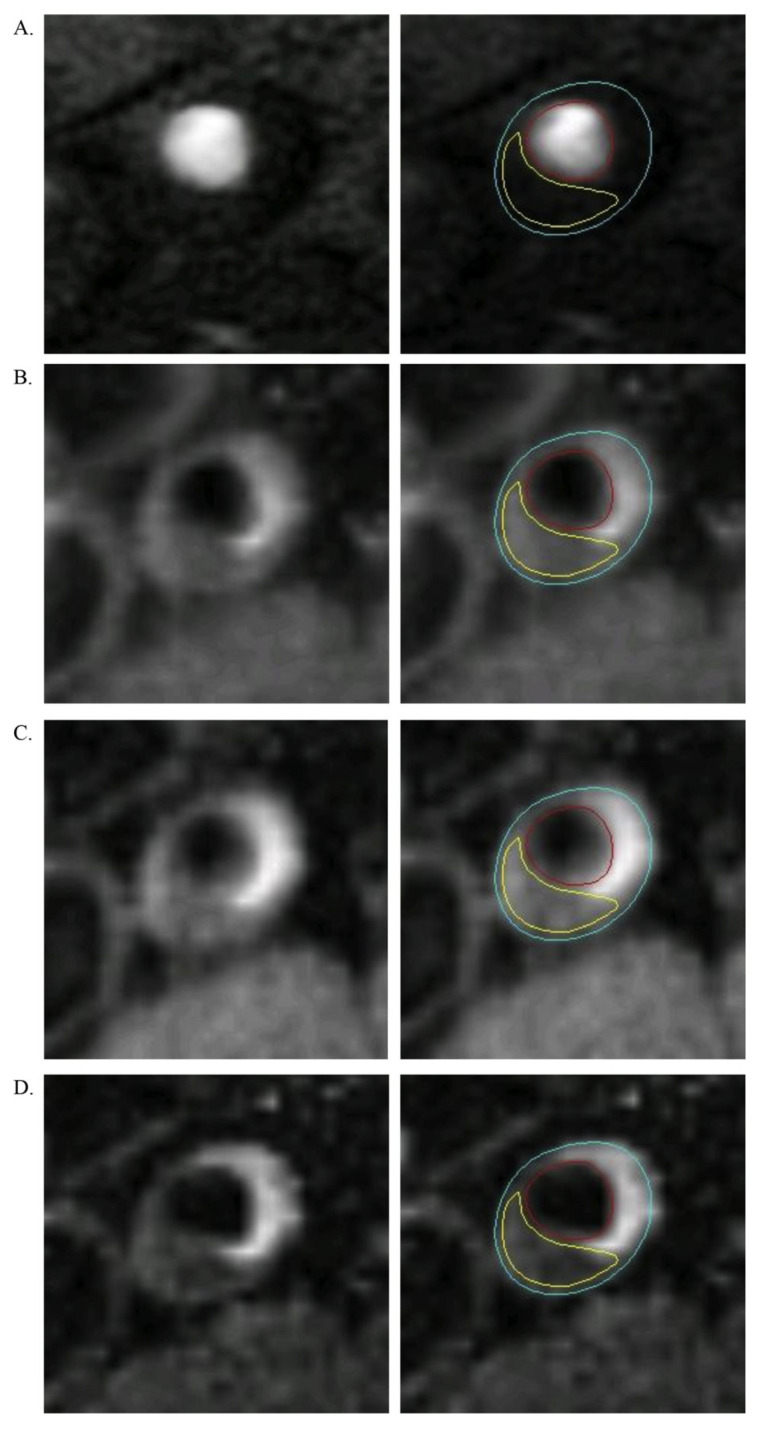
MRI presentation of superficial femoral artery plaque containing lipid-rich necrotic core. The imaging sequence is the time of flight (A); T1 (B); proton density (C); and T2 (D). The blue contour delineates the outer boundary of the SFA, the red contour delineates the lumen, and the yellow contour delineates the lipid-rich necrotic core. Image reprinted from Polonsky et al. [78].
